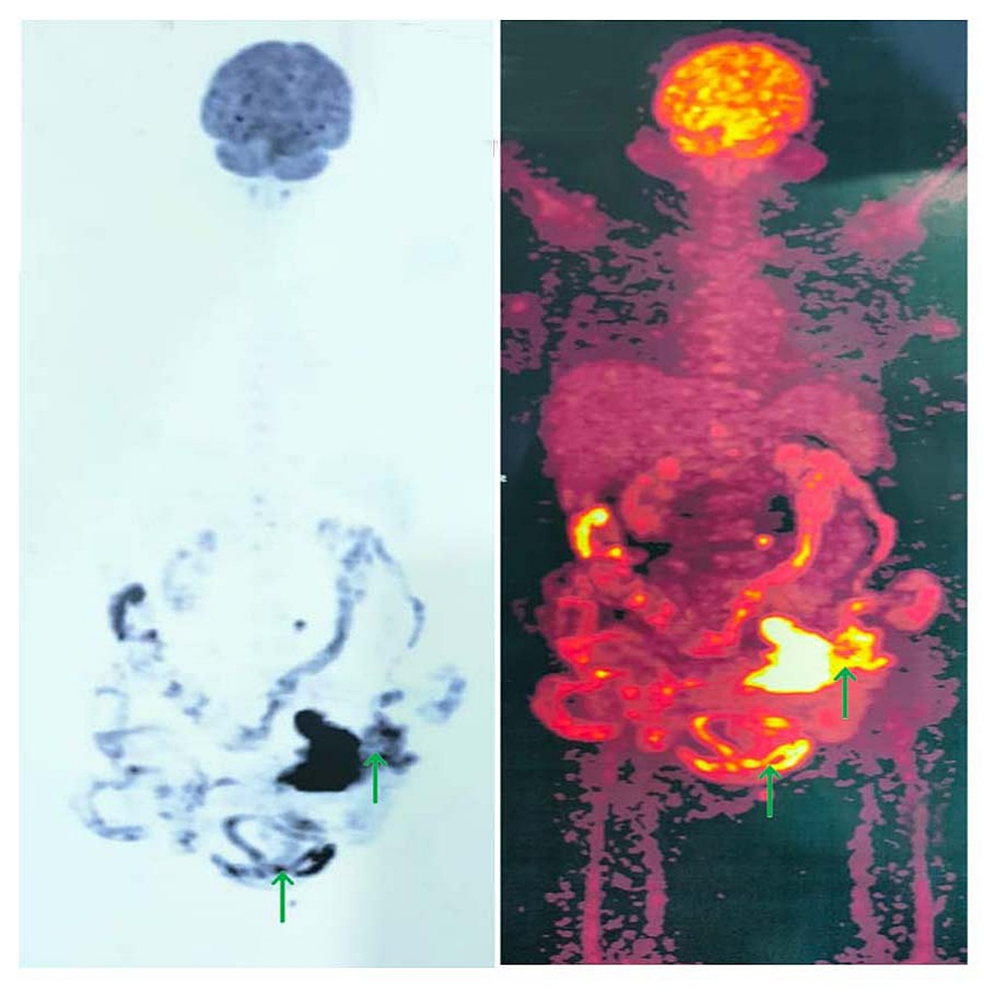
PET-CT scan showing physiological uptake of fluorodeoxyglucose (FDG) in the brain, head, neck, and thorax. FDG avid heterogeneously enhancing ill-defined lesion involving sigmoid colon, left superior-lateral aspects of urinary bladder, left lower anterolateral abdominal wall with adjacent muscles, and para-aortic and right inguinal lymph nodes suggestive of metastatic deposits. PET: positron emission tomography
